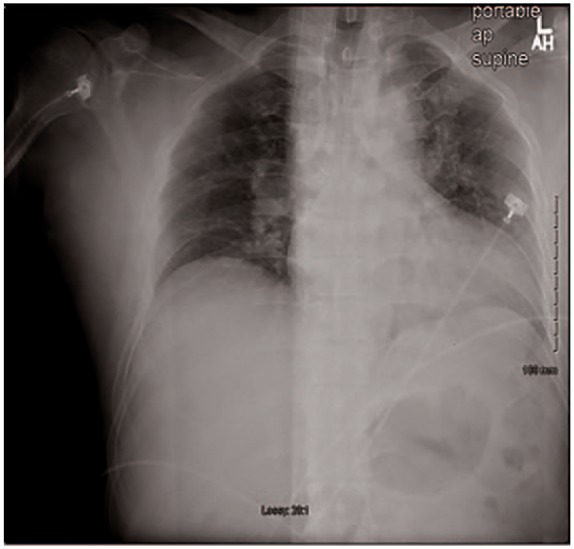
Chest X-ray on admission for case 2.
